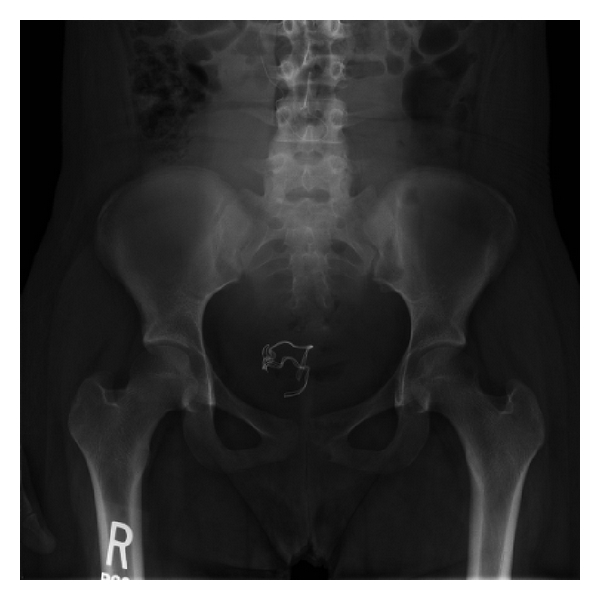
Postdelivery X-ray of the pelvis demonstrating two curvilinear triangular-shaped structures composed of 2 radiopaque threads in the vaginal/rectal area.
